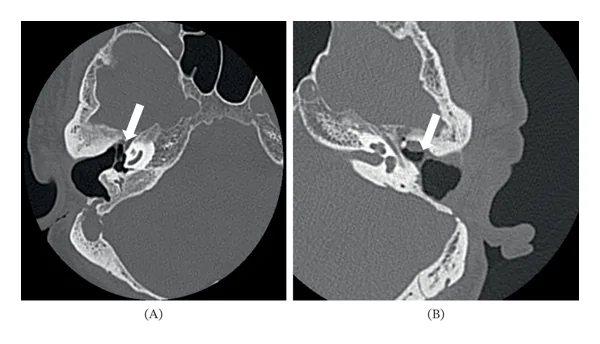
(A, B) Preoperative temporal bone computed tomography in Cases 1 and 2.
